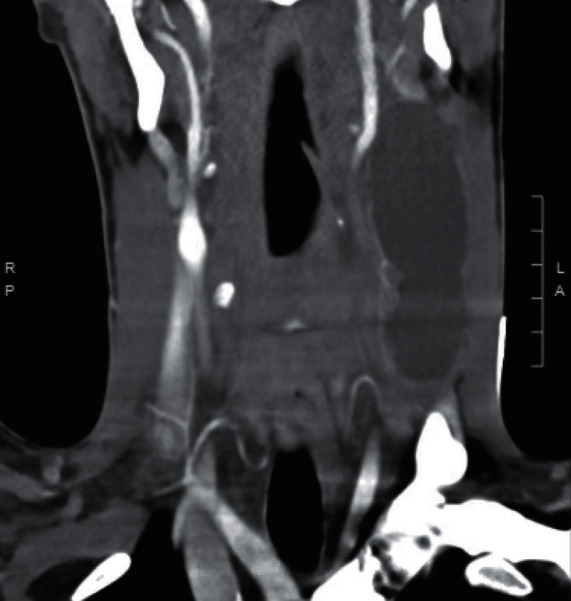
Computed tomography scan of the neck with contrast revealed a 4 cm × 8 cm × 3 cm cystic mass in the left neck occupying nodal levels 2–4 deep to the sternocleidomastoid muscle.
